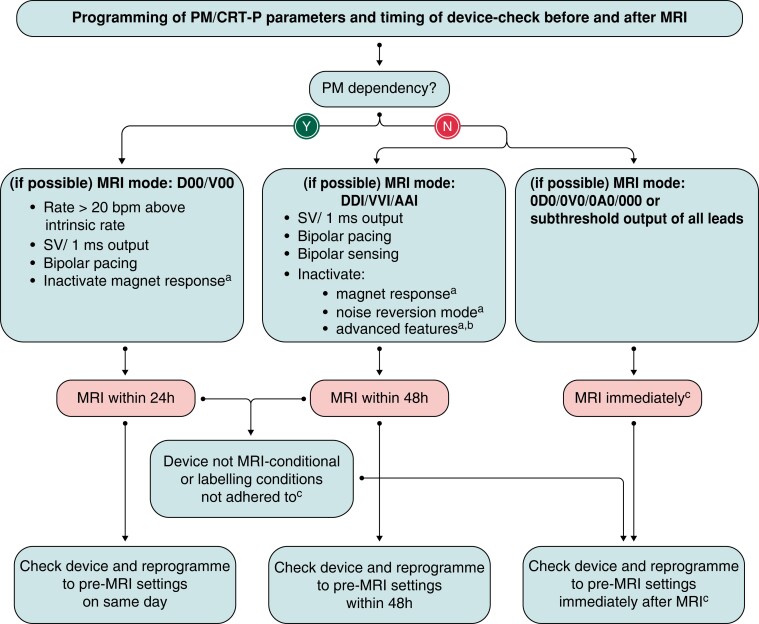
Programming of device parameters and timing of device check before and after MRI. From ref.^101^ AF, atrial fibrillation; MRI, magnetic resonance imaging. ^a^If available. ^b^Rate hysteresis; atrial anti-tachycardia pacing; premature ventricular complex and premature atrial contraction triggered pacing; AF therapies–rate smoothing; overdrive pacing; conducted AF response. ^c^In CIED with automatic MRI mode activation, the scan may be performed electively after the pre-scan follow-up and reprogramming after the intervention may not be necessary.
